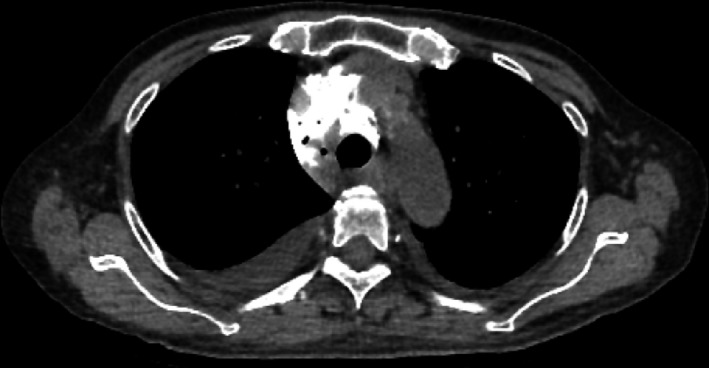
Chest CT after the injection of a contrast agent in the blood return line of the double‐lumen catheter. Contrast agent is visible in the mediastinum
